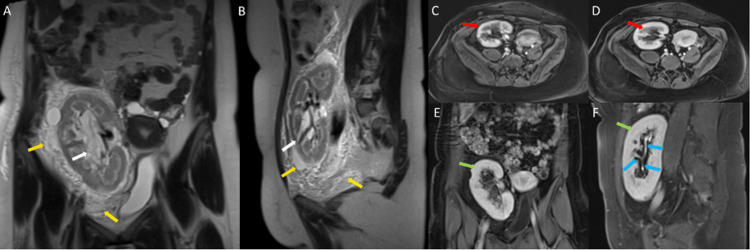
MRI of the abdomen with IV contrast: A) coronal T2W; B) sagittal T2W; and C) axial T1W fat saturated (fat sat) post-contrast arterial phase; D) axial T1W fat saturated post-contrast venous phase; E) coronal T1W fat sat post-contrast delayed phase; and F) sagittal T1W fat sat post-contrast delayed phase shows an enlarged transplanted kidney in the right iliac fossa with septated renal sinus cystic spaces (white arrows), perinephric fluid collection (orange arrows), and edema of the surrounding fat (yellow arrows). Post-contrast arterial and venous phases demonstrate adequate renal parenchymal enhancement (red arrows) and prompt excretion (green arrows) with opacification of the calyceal system (blue arrows) on delayed images. T2W: T2-weighted image; T1W: T1-weighted image
